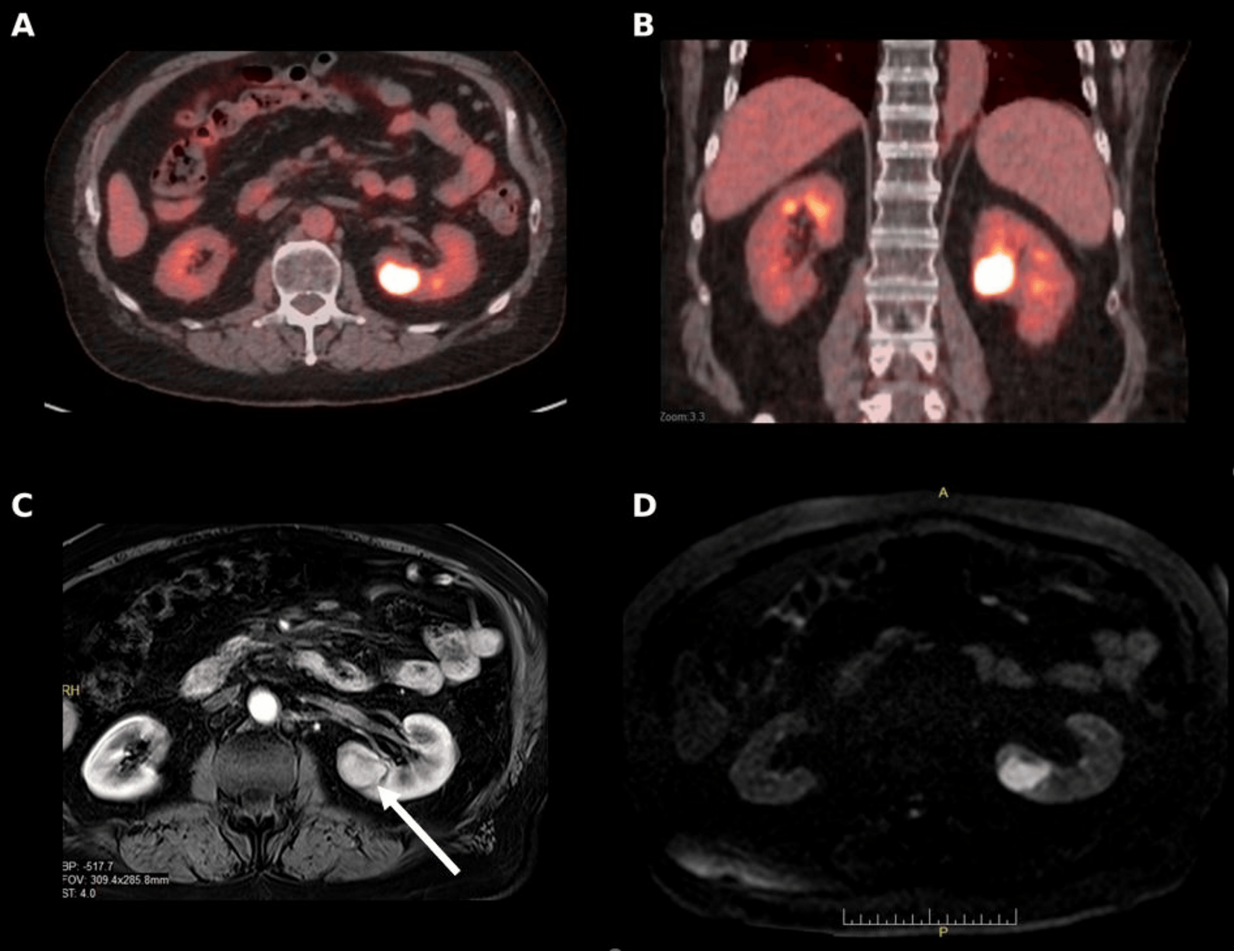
PET/CT and MRI demonstrating hypermetabolic left renal lesion (A) Axial positron emission tomography/computed tomography (PET/CT) demonstrating intense fluorodeoxyglucose (FDG) uptake in the mid-upper pole of the left kidney; (B) Coronal PET/CT confirming the hypermetabolic focus; (C) Contrast-enhanced T1-weighted magnetic resonance imaging (MRI) showing a 3.4 cm enhancing mass in the same region (white arrow); (D) Diffusion-weighted MRI demonstrating restricted diffusion at the lesion site, consistent with metastatic disease.
